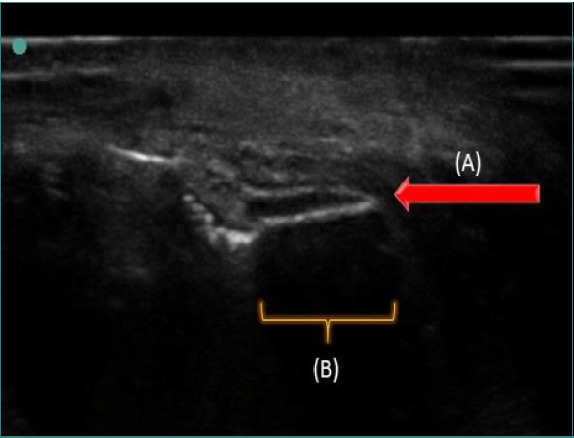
Intraoperative ultrasound. A: Foreign body as echogenic line, B: Posterior acoustic shadowing
